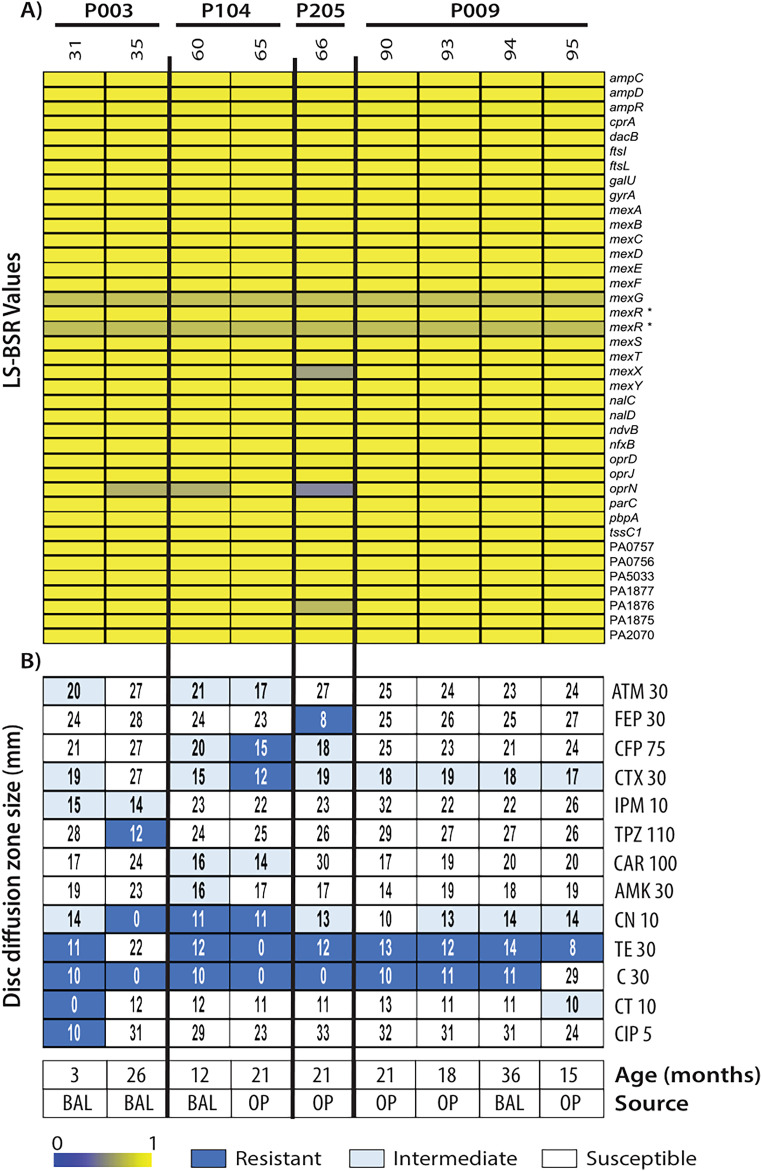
LS-BSR analysis of genes associated with antibiotic resistance and disc diffusion data. LS-BSR analysis of genes associated with antibiotic resistance (A) was coupled with disc diffusion data for several antibiotics (B): aztreonam (ATM 30), cefepime (FEP 30), cefoperazone (CFP 30), cefotaxime (CTX 30), imipenem (IPM 10), piperacillin-tazobactam (TPZ 110), carbenicillin (CAR 100), amikacin (AMK 30), gentamicin (CN 10), tetracycline (TE 30), chloramphenicol (C 30), colistin (CT 10), and ciprofloxacin (CIP 5). The numbers following the antibiotic abbreviations correspond to the antibiotic disc concentrations in micrograms. For reference, the patient age at the time of isolation and the isolation source are included. “*” indicates allelic variation.
